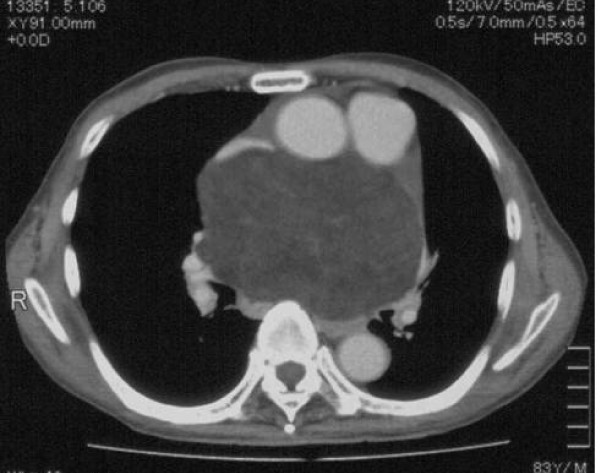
Computed tomography showed a large tumor behind the ascending aorta and pulmonary artery and compressing the right and left atria.
